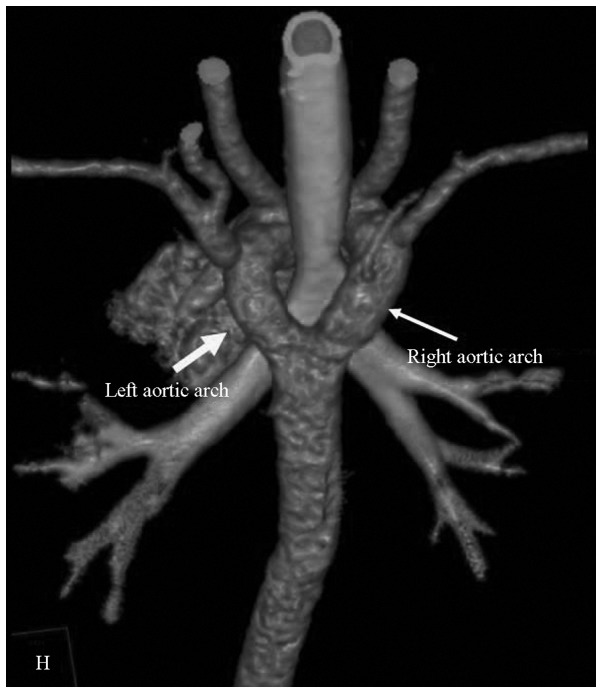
A nineteen-month-old male infant with DAA. The CT volume-rendered image shows that the aortic arch split into two arches surrounding the trachea (similar thickness of two arches). DAA, double aortic arch; CT, computed tomography.
